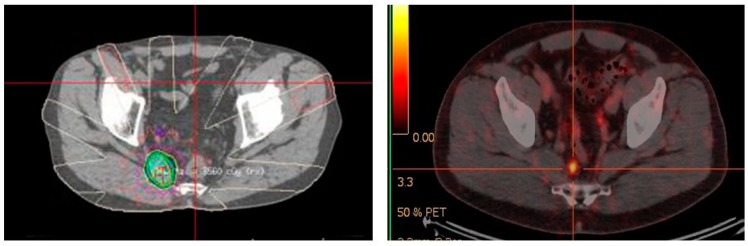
Targeted radiotherapy to recurrent ^68^Ga-PSMA avid right pre-sacral lymph node. June 2015.
